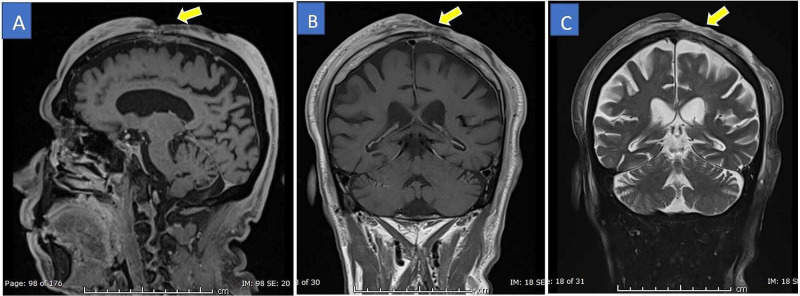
Brain MRI – sagittal T1-weighted image (A), coronal T1-weighted image (B), and coronal T2-weighted image (C) The images show an extensive bifrontal-parietal scalp lesion that is infiltrating the left frontal and parietal bone, passing through the calvarium, infiltrating the dura, and causing dural enhancement MRI: magnetic resonance imaging
